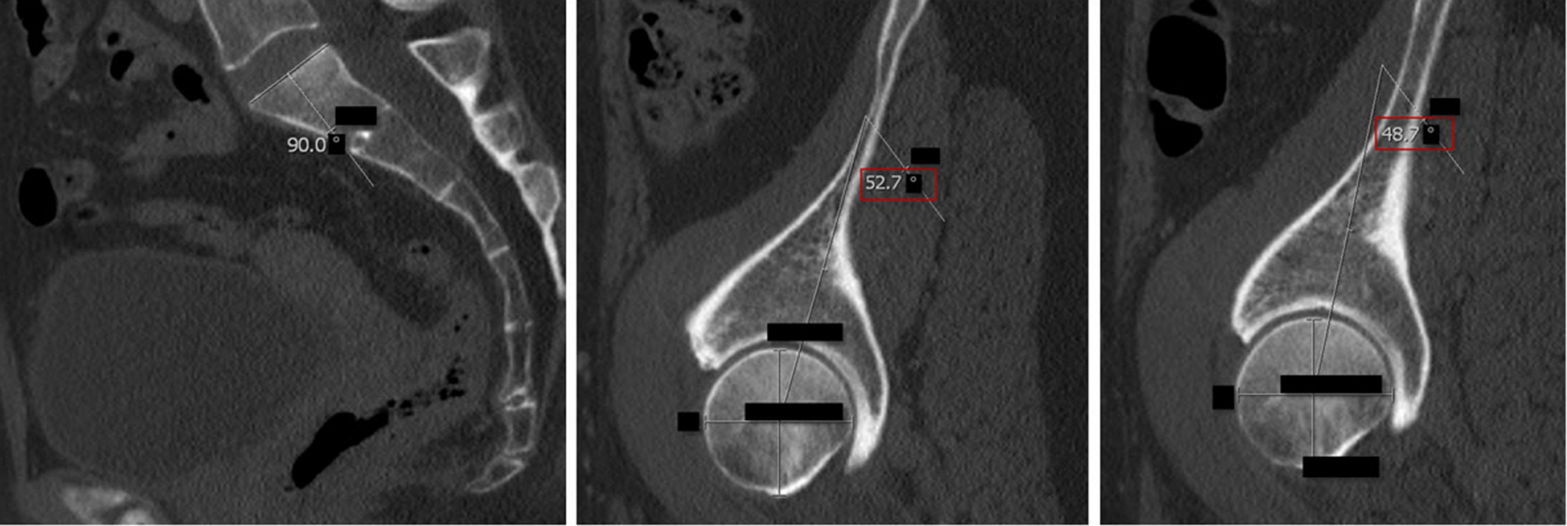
Pelvic Incidence (PI): The PI was determined on sagittal CT planes of the pelvis. A line was drawn along the axis of the superior end plate of S1 (left image). Then, originating at the center of this axis, an orthogonal line was drawn (left image). Secondly, the middle of the femoral head was determined by the intersecting point of a vertical and horizontal line within the femoral head (middle and right image). Finally, a line was drawn from the middle of the each femoral head to the center of the superior end plate axis and the angle was measured in regard to the orthogonal line originating at this point (middle and right image). The red box indicates the PI. The blacked out numbers were disregarded because they were created automatically by our software and contained irrelevant information. In order to acquire the superposition of the two femoral heads, left and right, the PI was measured for both sides and the mean was stated.
